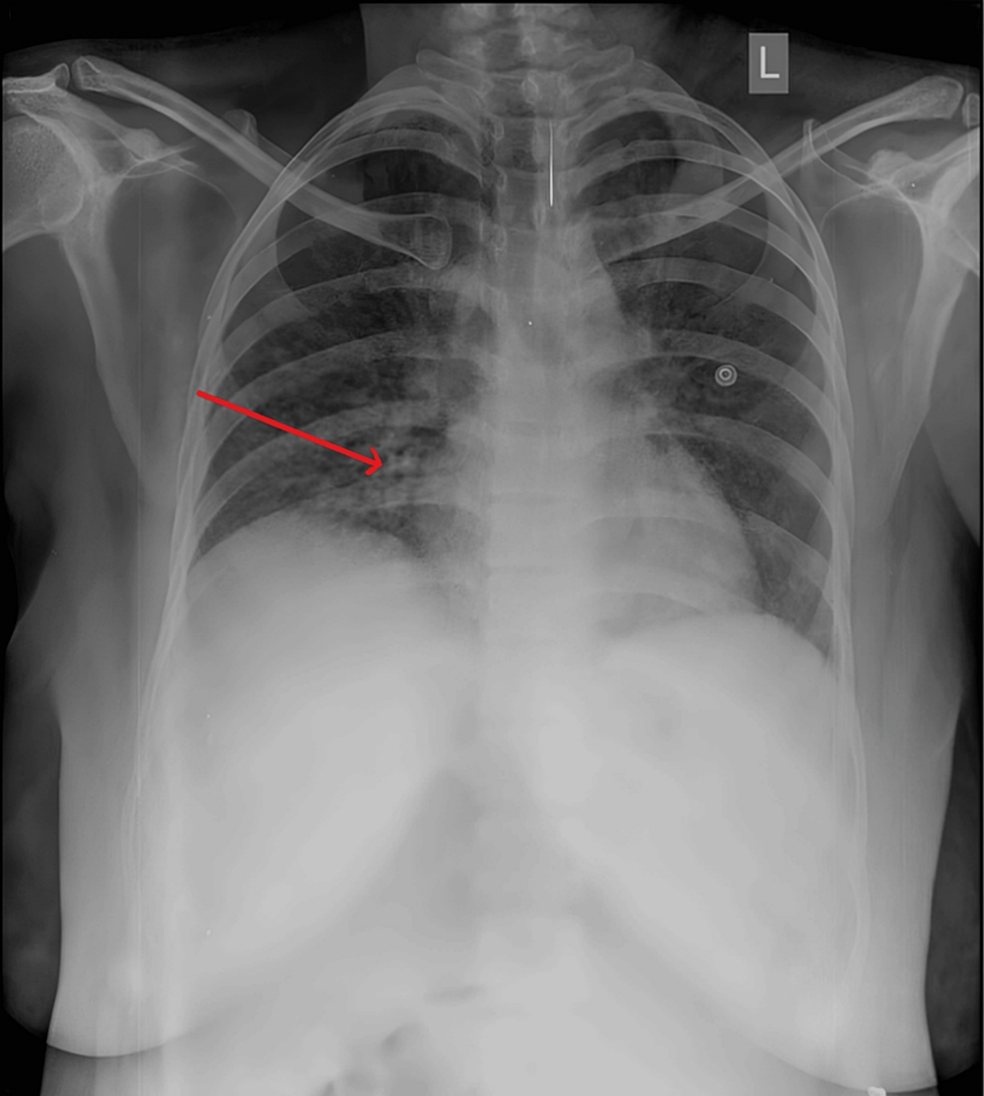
Chest X-ray suggestive of early signs of pneumonia.
